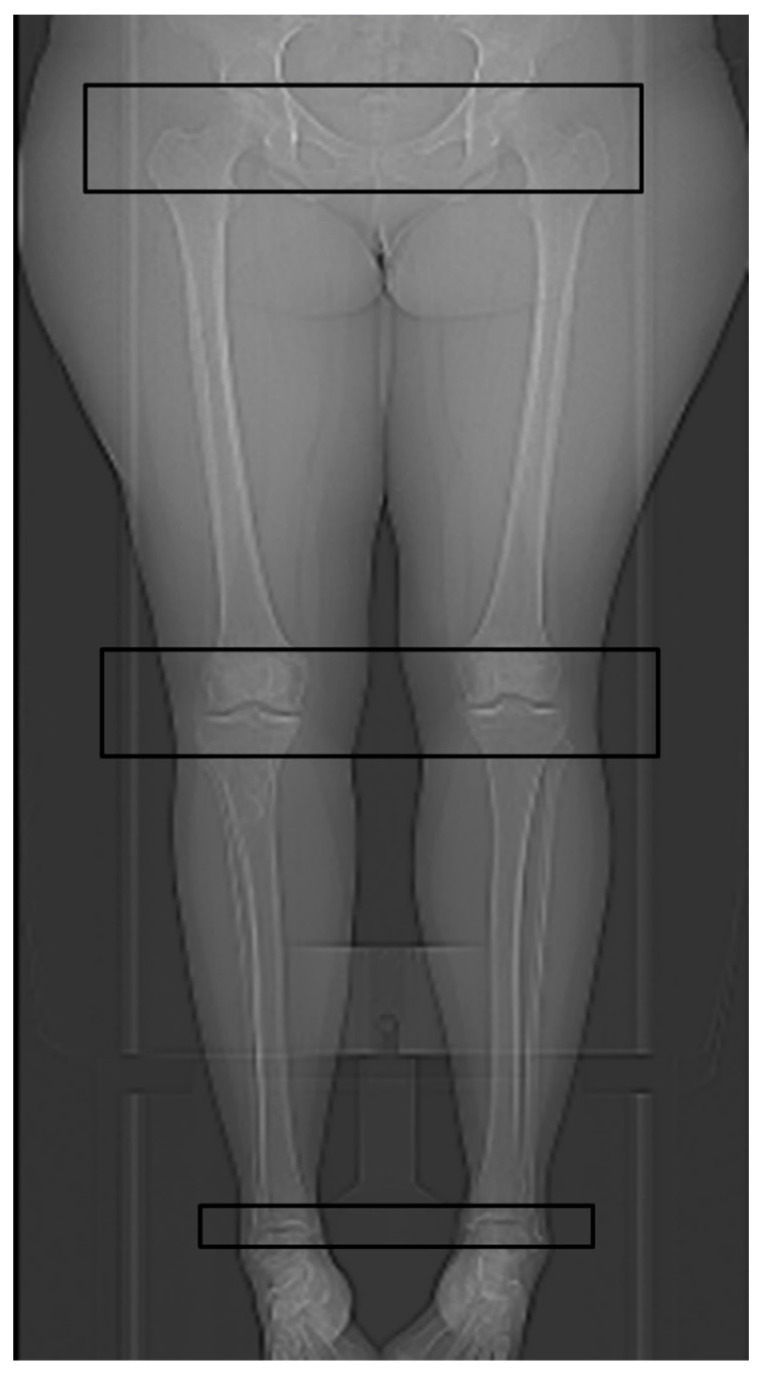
Anterior-posterior (AP) scout view with the marked scan length for the hip region, the knee region, and the ankle region according to the standard operating procedure (SOP) for the newly introduced ULD protocol for CT-torsion measurement of the lower limb.
